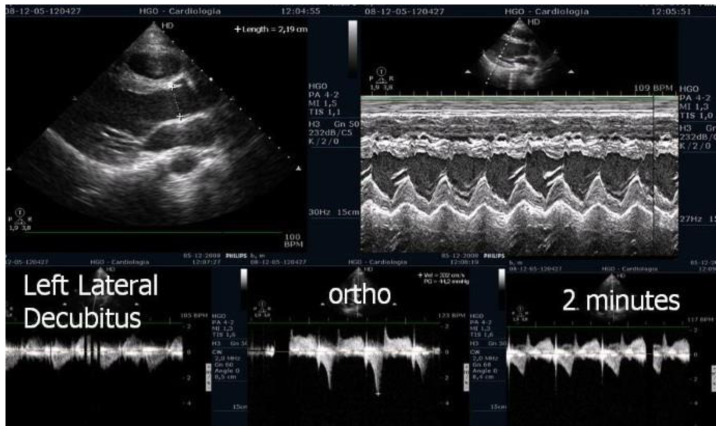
Echocardiography before exercise in a symptomatic athlete in the left lateral decubitus position and in the orthostatic position before and at the beginning of exercise [3].
